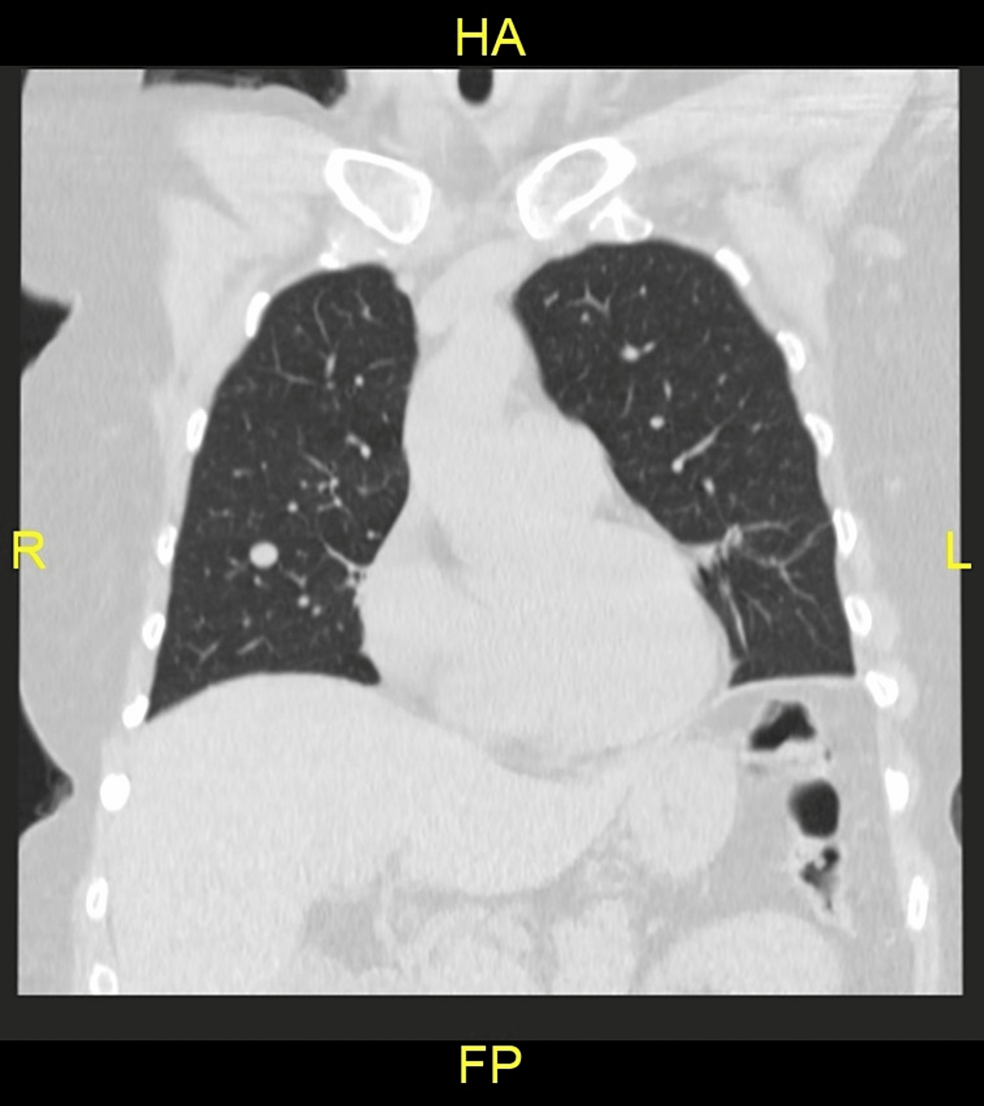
Chest CT findings of a 56-year-old female patient: coronal non-contrast CT section 1 cm diameter right middle lobe nodule
